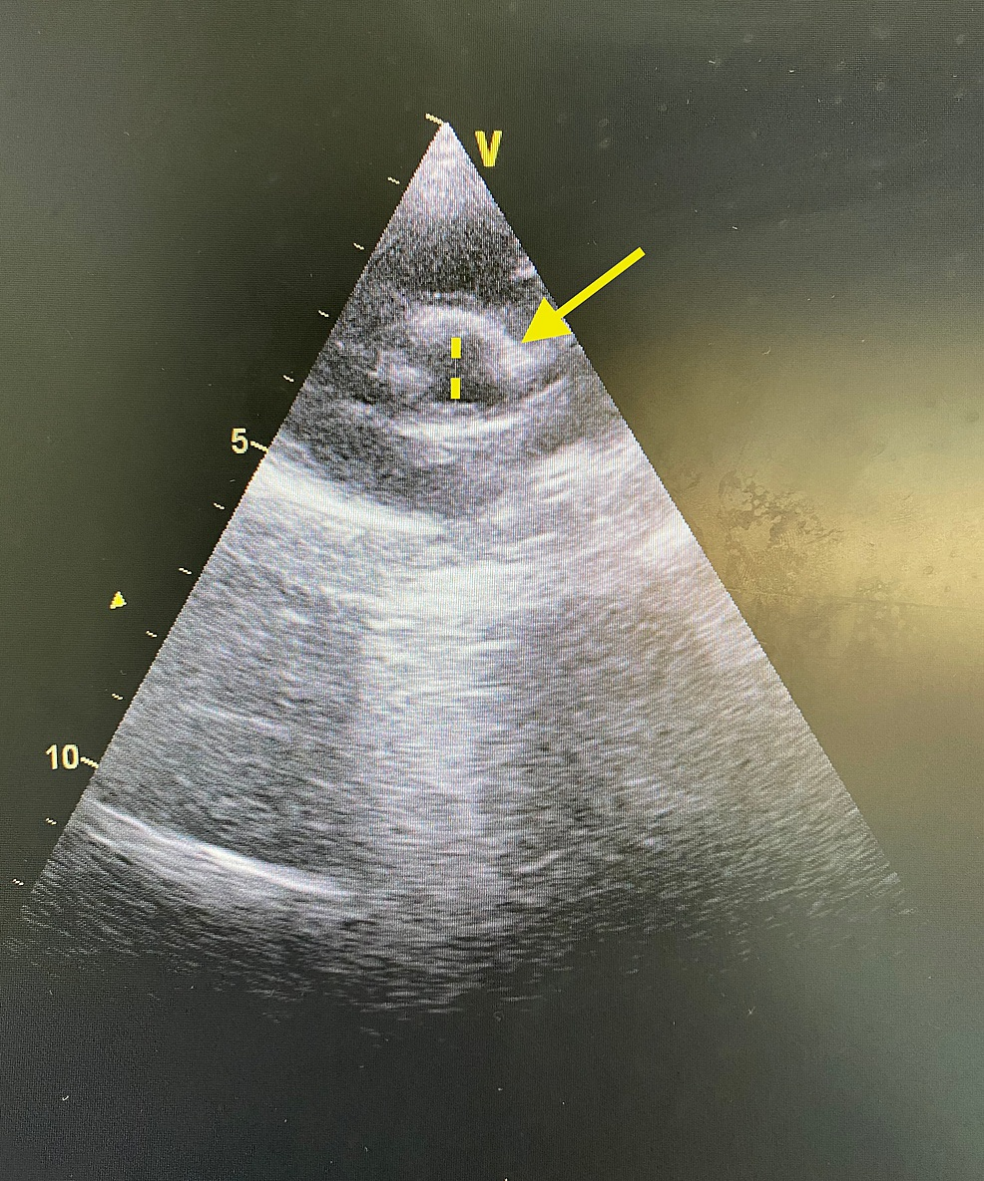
Echocardiogram showing coronary artery prominence with mild dilatation.
